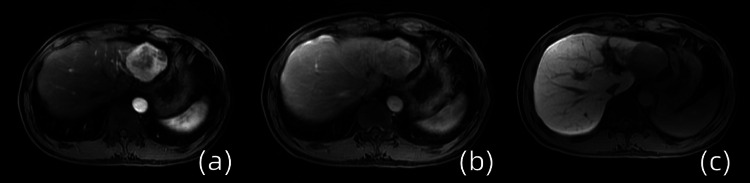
Enhanced magnetic resonance imaging (a) The arterial phase revealed the presence of heterogeneous hyperenhancement. (b) The signal intensity showed a reduction during the venous and transitional phases. (c) The signal intensity decreased during the liver-specific phase
